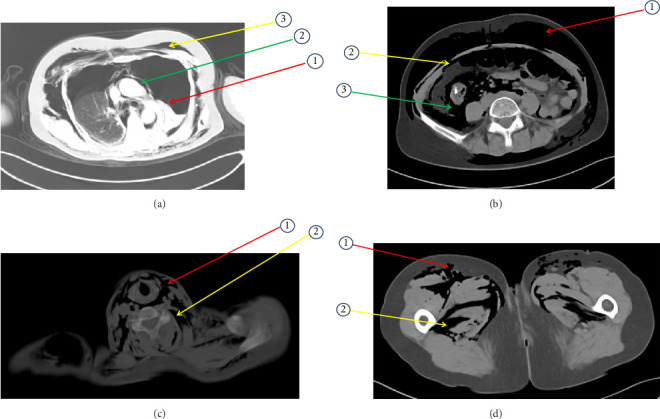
(a) Axial CT (lung window) showing: ① bilateral pneumothorax with near-total collapse of the left lung (red arrow); ② pneumomediastinum anterior to the ascending aorta (green arrow); ③ extensive subcutaneous emphysema in the left chest wall (yellow arrow). (b) Axial CT (abdominal window) demonstrating: ① extensive subcutaneous emphysema in the anterior abdominal wall (red arrow); ② pneumoperitoneum (yellow arrow); ③ pneumoretroperitoneum in the bilateral perirenal spaces (green arrow). (c) Axial CT of the neck showing: ① subcutaneous emphysema in the platysma and superficial fascia (red arrow); ② gas dissecting along the strap muscles and deep cervical fascia (yellow arrow). (d) Axial CT through the upper thighs showing: ① extensive subcutaneous emphysema (red arrow); ② gas extending along the fascial planes in the anterior and medial compartments of the thigh (yellow arrow).
